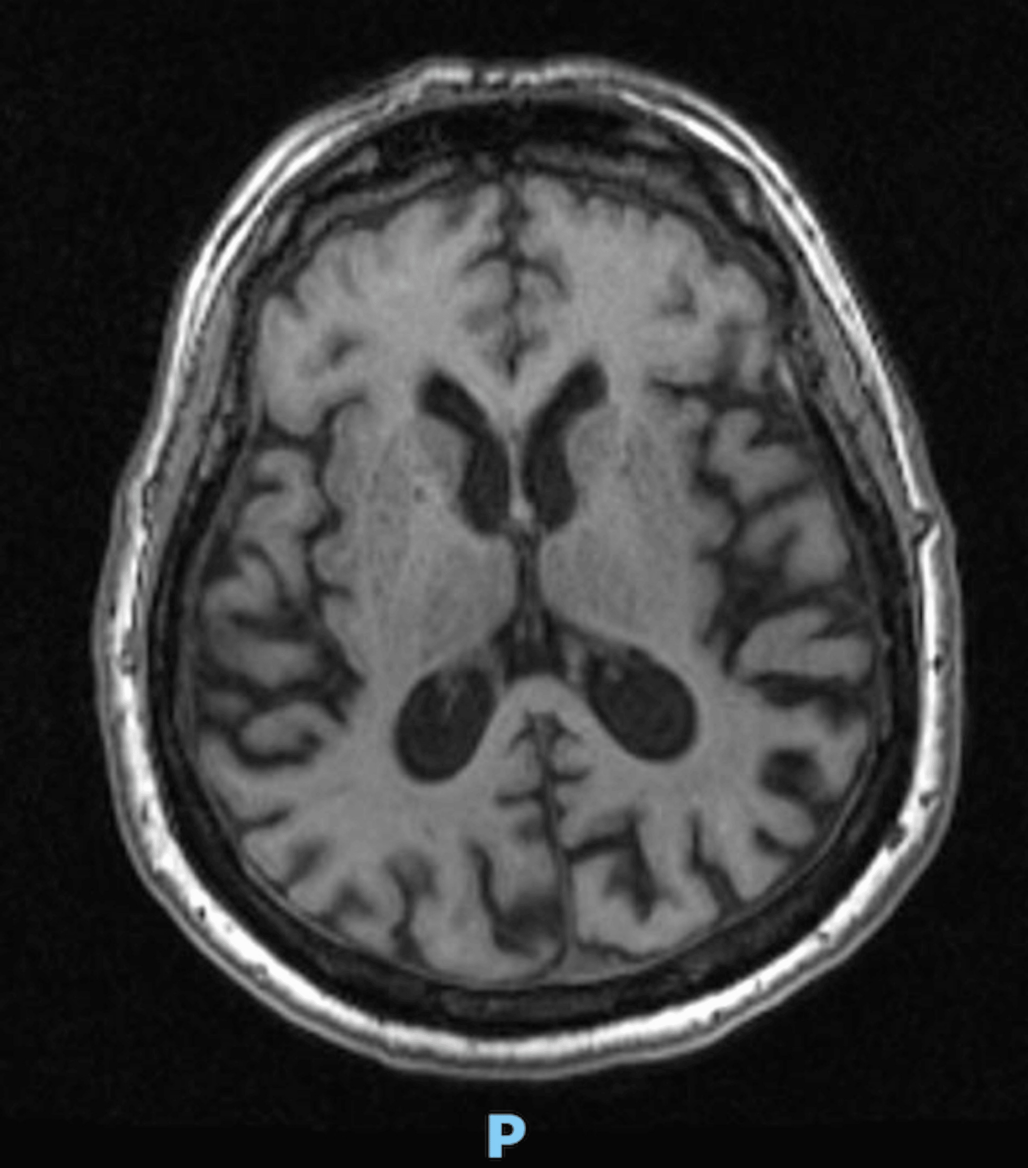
September 2022: MRI head showing progressive age, excessive reduction in global cerebral volume
